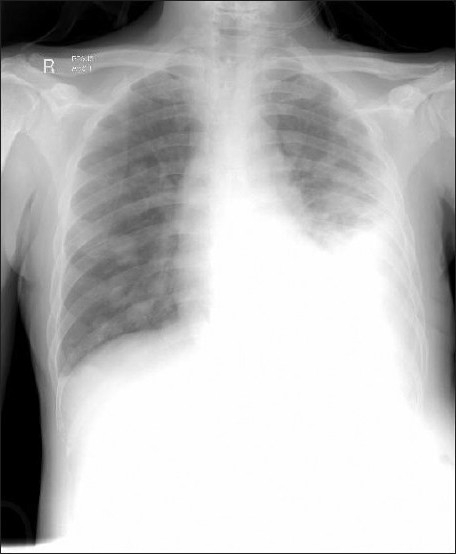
Chest radiograph taken 3 months postoperatively showing lung metastasis and a moderate left pleural effusion. Preoperative chest radiograph was normal
